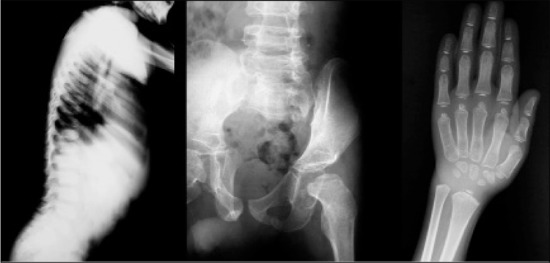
X-ray films demonstrating beaking of both thoracic and lumbarvertebrae, spina bifida at L5 vertebrae, generalized epiphysealmetaphysealdysplasia and osteopenia of the skeletal system
